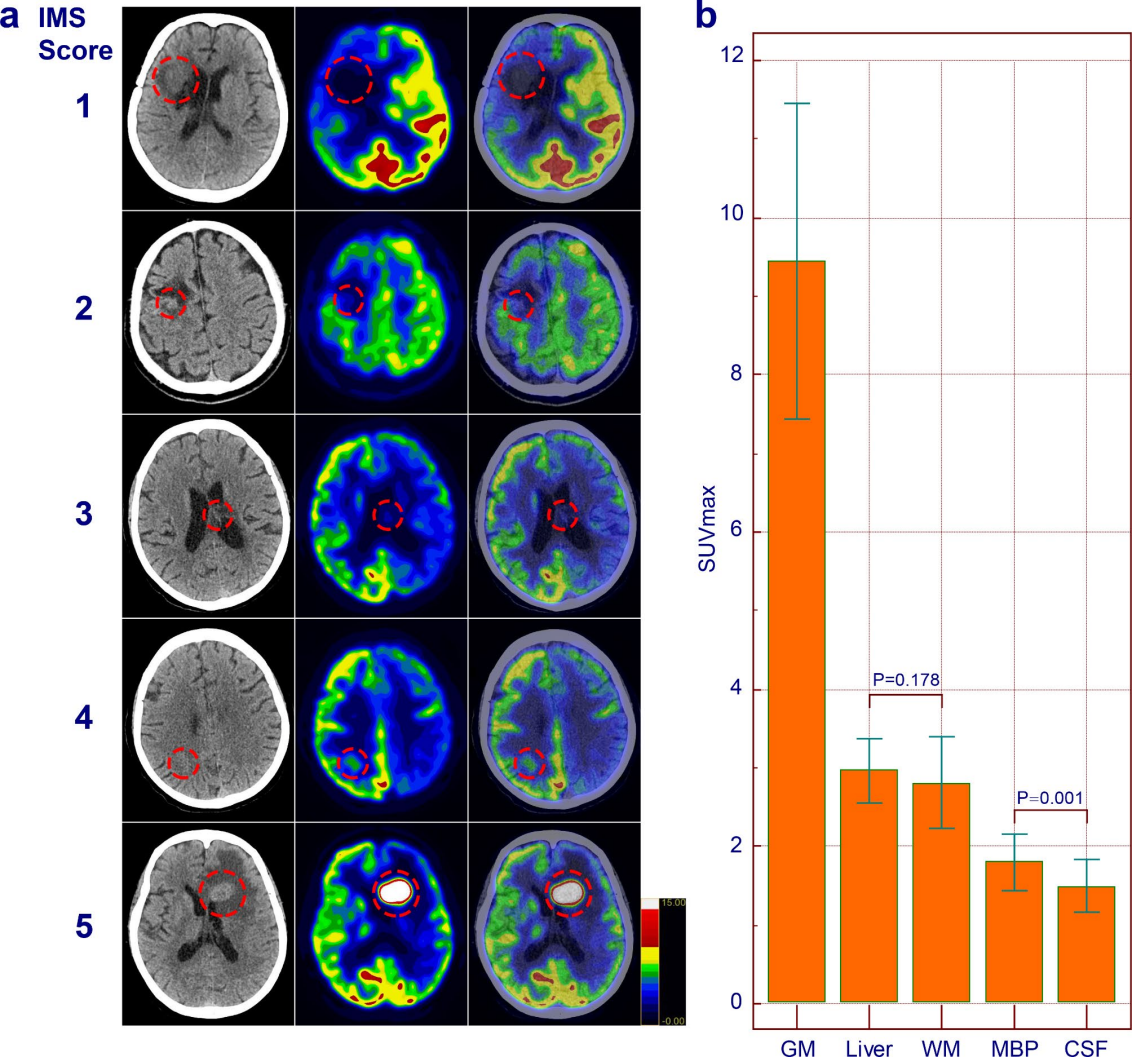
a Examples of the IMS scale based on FDG metabolism in normal intracranial structures (IMS 1, no residual uptake; IMS 2, residual uptake > cerebrospinal fluid but < cerebral white matter; IMS 3, residual uptake similar to cerebral white matter; IMS 4, residual uptake > cerebral white matter but < gray matter; IMS 5, residual uptake > gray matter and/or the presence of new lesions). b Comparison of the SUVmax of the reference normal structures for the IMS and DS scales. IMS, intracranial metabolism score; DS, Deauville score; GM, gray matter; WM, white matter; CSF, cerebrospinal fluid; MBP, mediastinum blood pool
